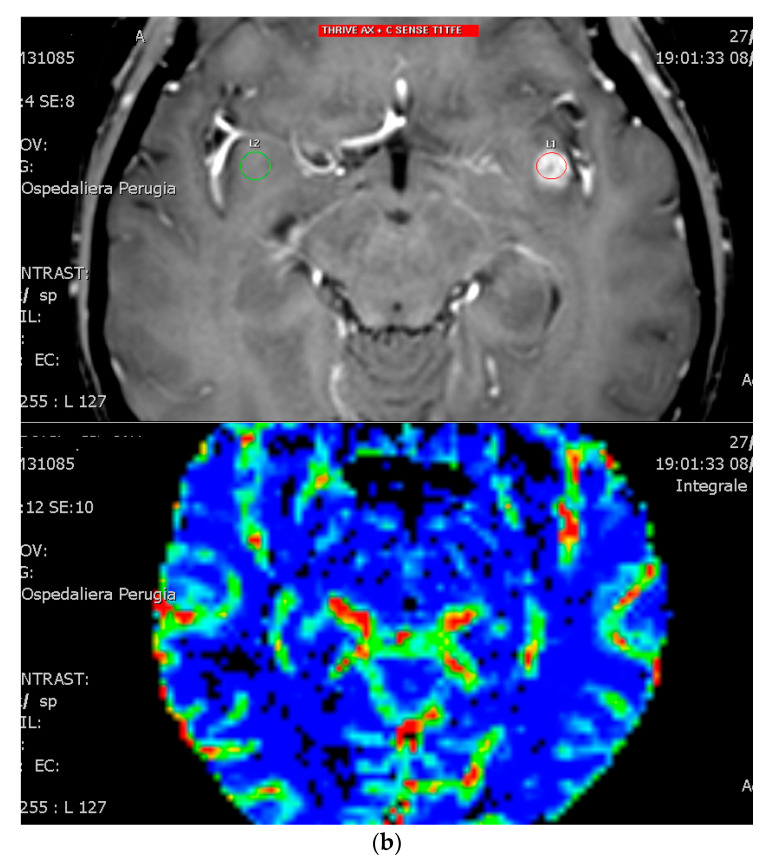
(a) Contrast enhanced T1-weighted magnetic resonance imaging (MRI) showing a suspected in-field recurrence one year after SRS (20 Gy) for a breast cancer metastasis in the left insular cortex; (b) the perfusion study is suggestive of disease progression because of relative cerebral blood volume (rCBV) increase due to the neo-angiogenesis within the insular cortex tissue.
